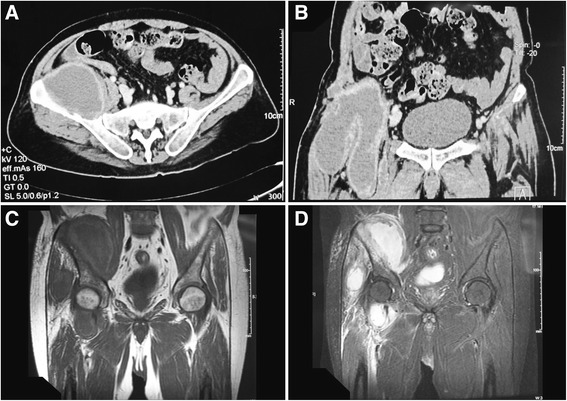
Contrasted CT scan and non-contrasted MRI scan of pelvic cavity: a Axial view of CT scan. b Coronal view of CT scan. c Coronal view of MRI T1-weighted fast spin echo sequence. d Coronal view of MRI short TI inversion recovery sequence. Both CT and MRI scan revealed a large right iliac abscess
